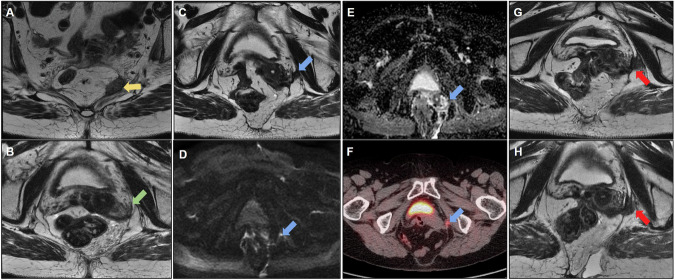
A Follow-up of a patient who underwent rectosigmoidectomy with coloanal anastomosis, revealing recurrence in the pelvic sidewall (yellow arrow). B The patient underwent abdominoperineal amputation with resection of the recurrent pelvic sidewall, showing usual postoperative changes (green arrow). C During follow-up, there was a progressive increase in serum levels of the tumor marker (CEA), and a nodular formation with intermediate signal intensity on T2-weighted imaging was visualized in the left pelvic sidewall (blue arrow), with diffusion restriction with a high signal on diffusion-weighted imaging (DWI) (D), and a low signal on ADC (E). F Positive fluorodeoxyglucose (FDG) uptake on positron emission tomography (PET/CT) (blue arrow), suspicious for neoplastic recurrence. G, H Follow-up MRI demonstrating growth of the neoplastic tissue (red arrow)
